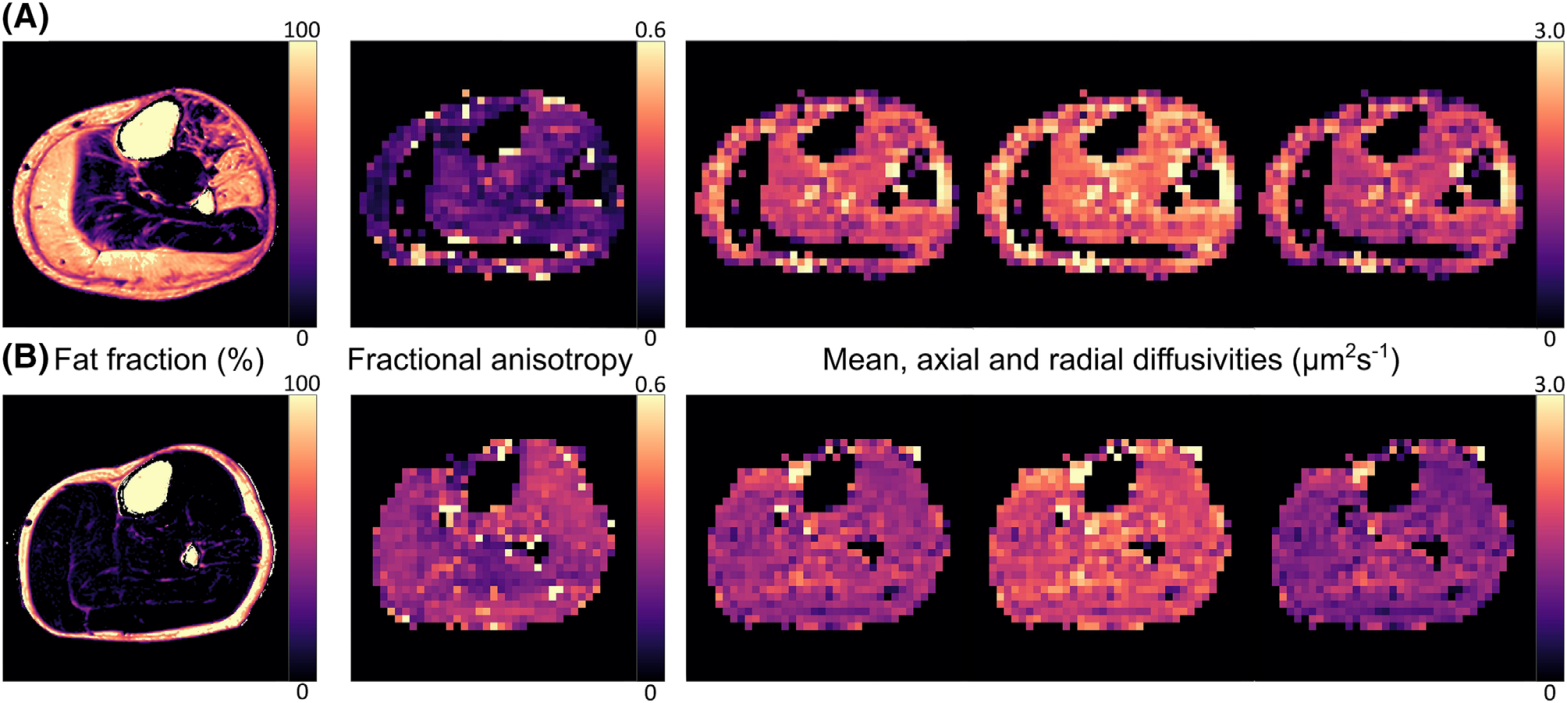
Representative lower leg ‘Dixon’ chemical‐shift‐based water‐fat separation images and stimulated‐echo diffusion‐tensor magnetic resonance imaging (STE‐DT‐MRI) parameter maps from a 59‐year‐old Becker muscular dystrophy (BMD) patient (A) and a 58‐year‐old male healthy control (B). STE‐DT‐MRI data were acquired with a diffusion time of 330 ms. The BMD patient shows severe fat replacement in the gastrocnemius medialis and lateralis and in the peroneus longus and extensor digitorum longus muscles. This leads to signal voids in these regions in the DT‐MRI data, which were acquired with comprehensive fat suppression.^20^
